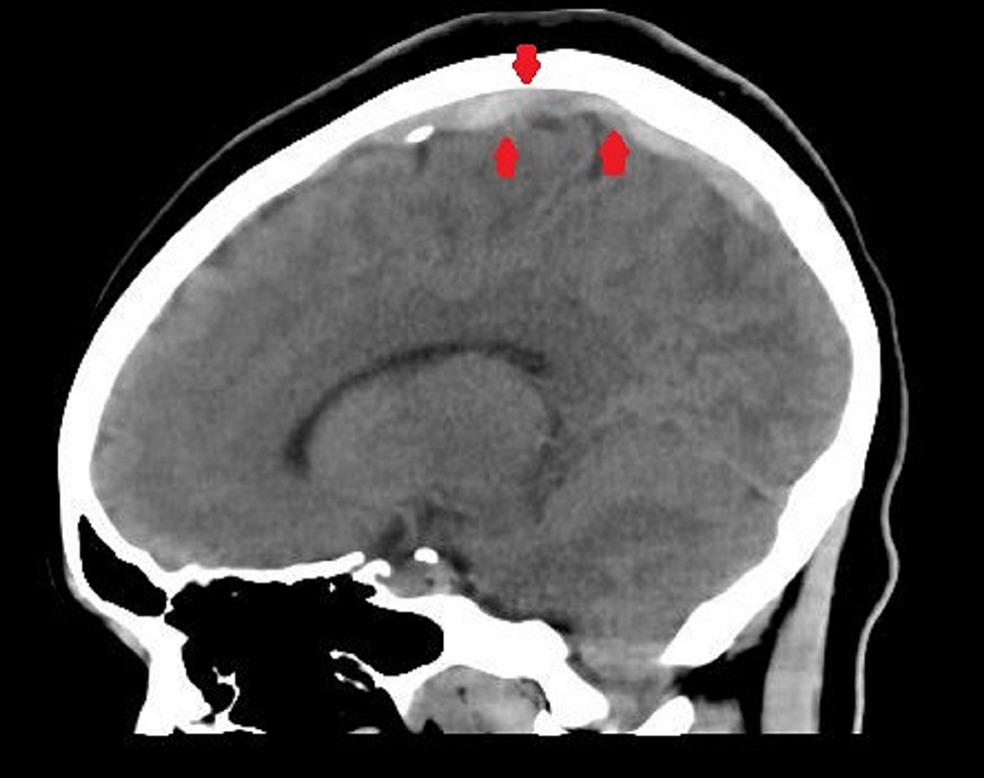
A sagittal section of brain CT scan showing a dense appearing superior sagittal sinus suspicious for thrombosis (arrows)
